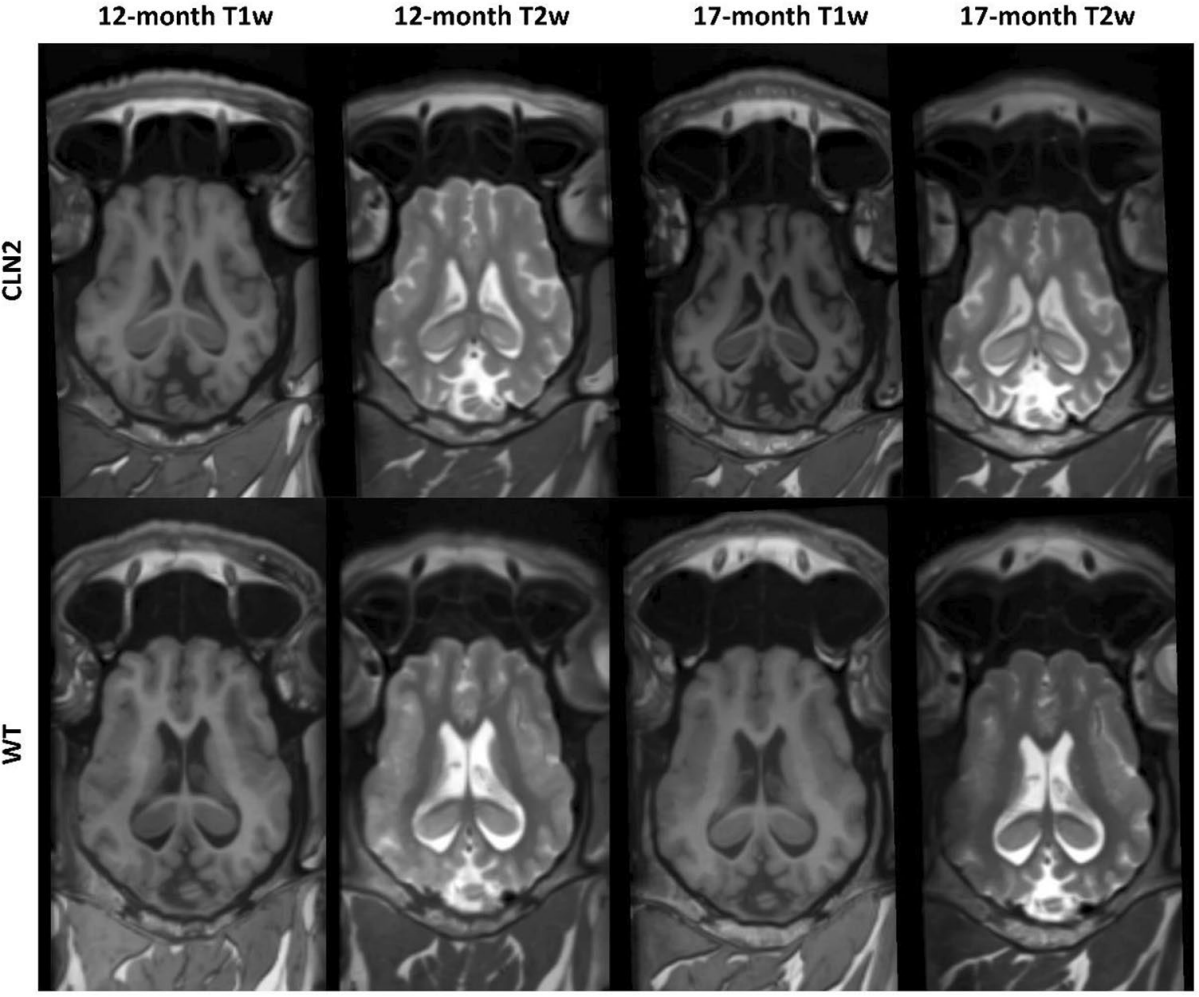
Exemplary T1-weighted (T1w) and T2-weighted (T2w) MRI data from a *CLN2*^*R208X/R208X*^ miniswine at 12-months-of-age (top left) and 17-months (top right), and a wild type (WT) comparator (bottom). Images were aligned using anterior commissure, posterior commissure, and basal pons, so that equivalent axial slices were taken from each time point.
